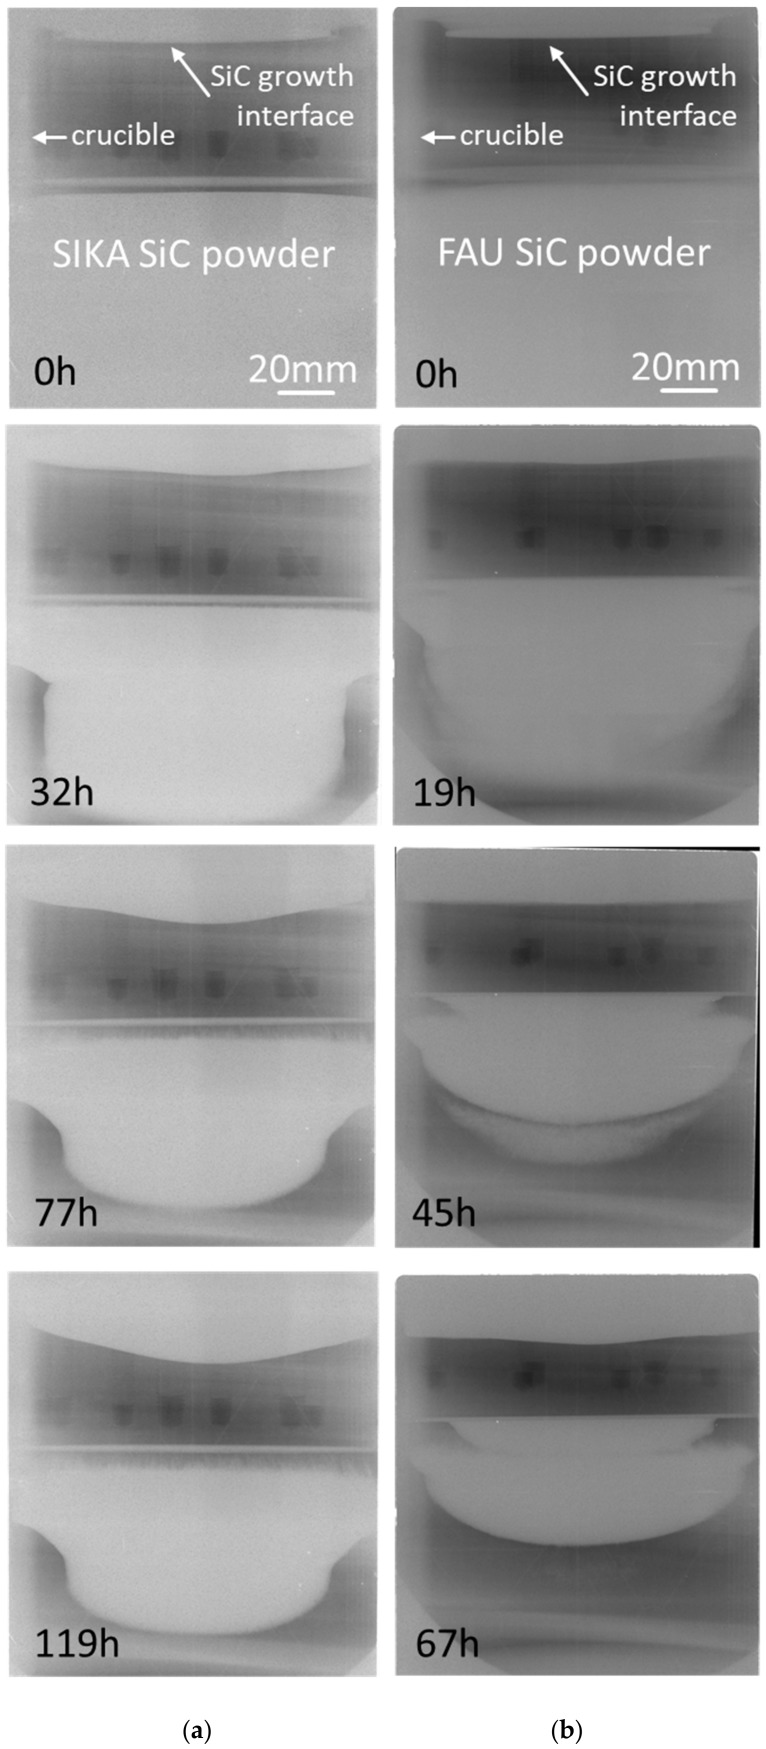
Series of 2D in situ X-ray visualization of the growth process of the 100 mm PVT growth process: (a) SiC powder from SIKA, and (b) reference SiC powder from FAU.
